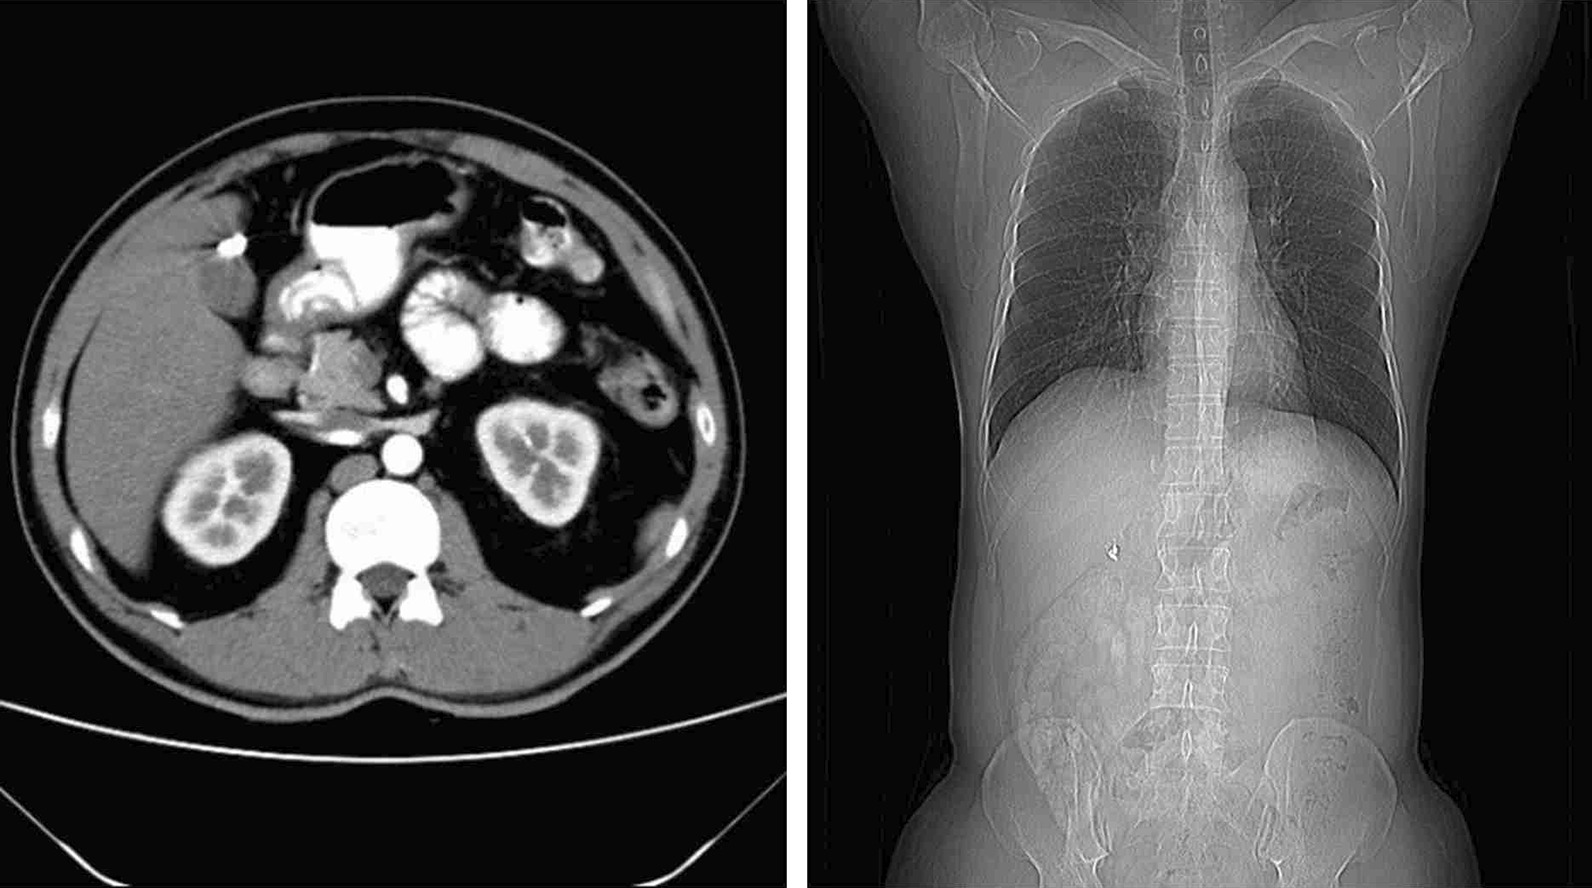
CT indicated the retention of titanium clip hemostatic clamps 12 months after surgery
